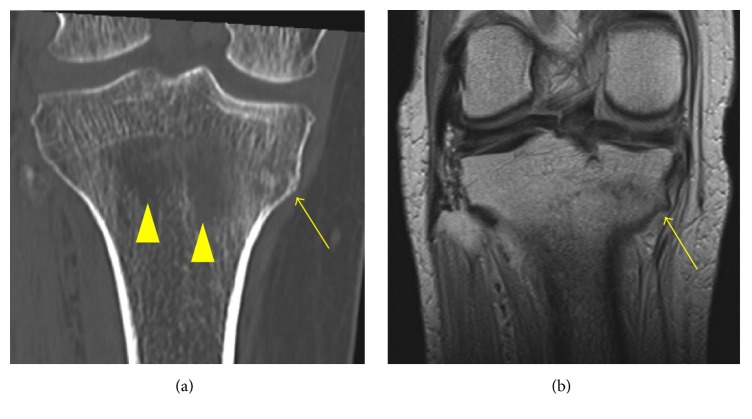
(a) Coronal CT imaging of right knee shows irregularity of cortex in the proximal medial tibia (yellow arrow) with trabecular bone resorption in the central part of the proximal tibia (yellow arrow heads), which indicates osteomalacia. (b) Coronal T1 weighted MR imaging of right knee shows linear low intensity lesion at the proximal medial tibia (yellow arrow), which indicates insufficiency fracture.
